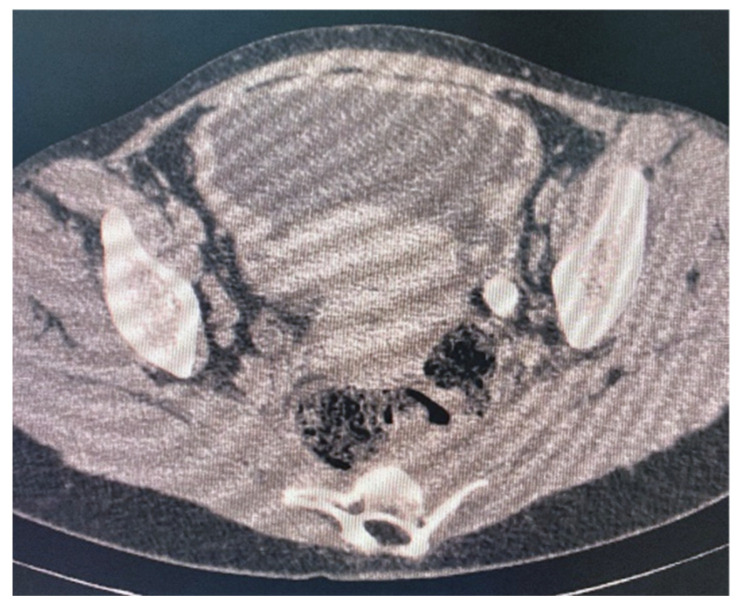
Uro-CT showing an infiltrative contrast enhancing mass located at the level of the posterior bladder wall.
